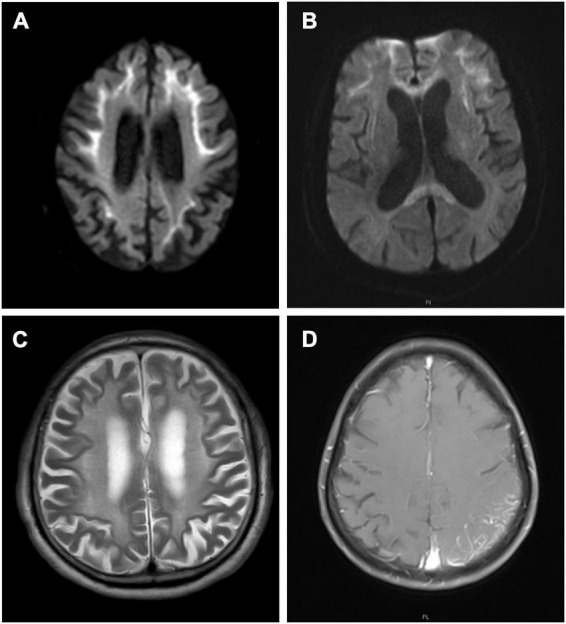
Cerebral magnetic resonance imaging (MRI) features of neuronal intranuclear inclusion disease (NIID) patients. Diffusion weighted imaging (DWI) showed curve-like hyperintensity along the corticomedullary junction (A); DWI also showed lesions in the corpus callosum (B); T2-weighted showed symmetrical white matter lesions (C); Contrast T1-weighted showed the left temporal occipital cortical edema with enhancement (D).
